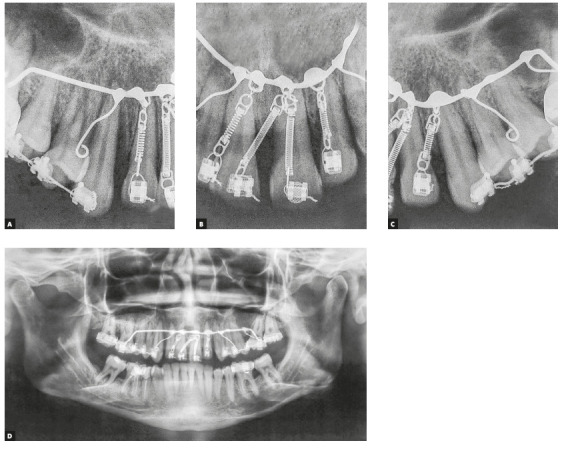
A-C) Periapical radiographs of the activated palatal arch in the patient. D) Panoramic radiograph of the activated palatal arch in the patient.
